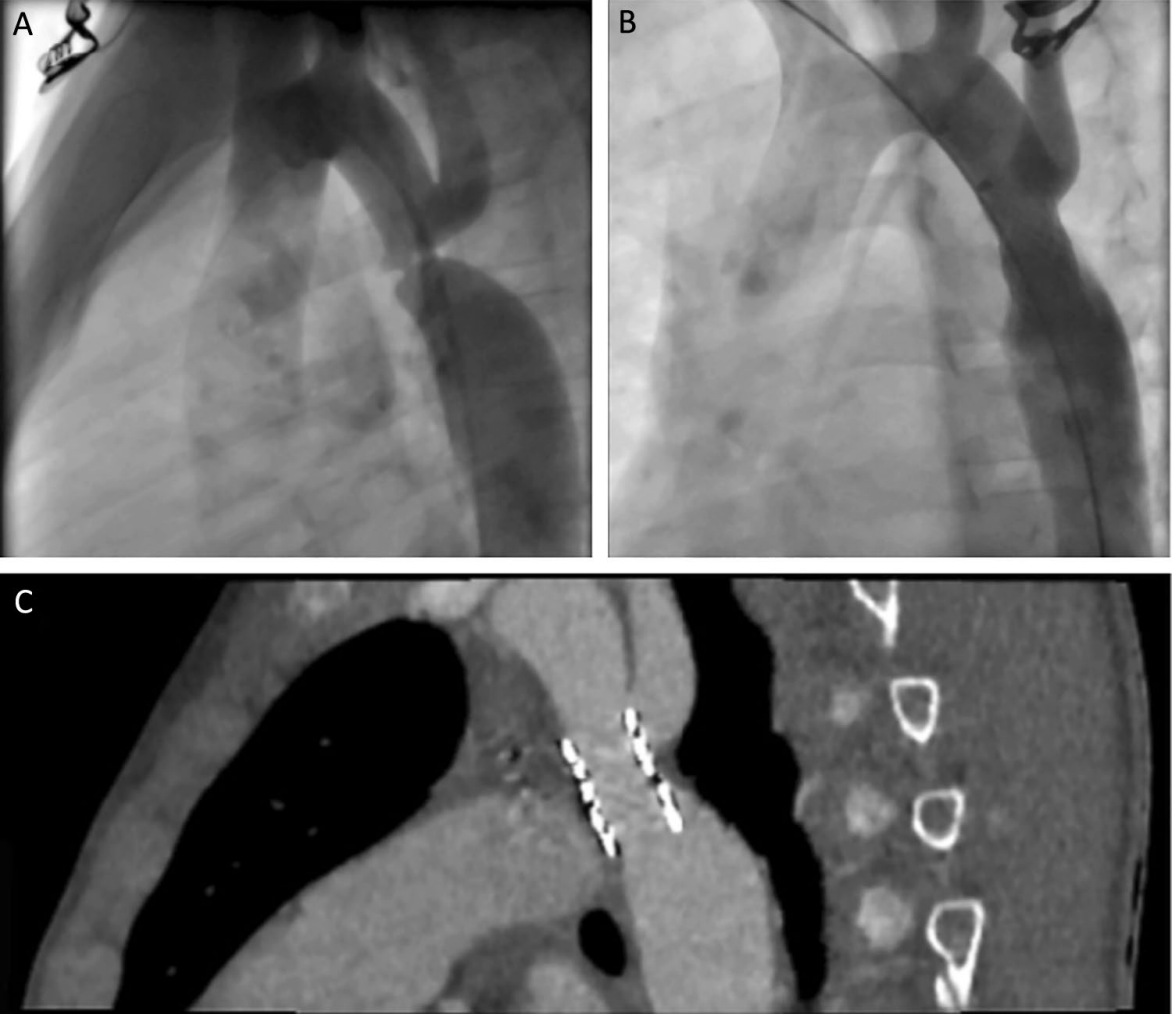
Aortograms and CT of a case using a BeGraft stent. A Lateral aortogram shows the left subclavian artery arising posteriorly immediately followed by a severe CoA. B Lateral aortogram showing a well-expanded 12 mm × 19 mm stent in position with matching vessel calibre. C CT performed 3 months after stent implanting demonstrating no aortic wall complications and preserved stent integrity. Although the stent edge partially covered the origin of the left subclavian artery the patient remained asymptomatic
